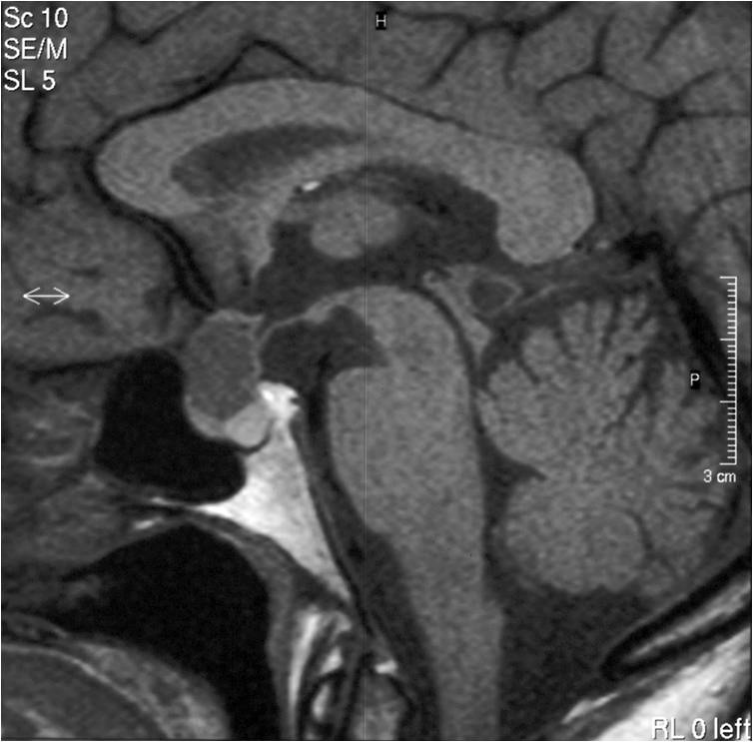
MRI, T1 weighted images, saggital section. A cystic lesion can be seen above the pituitary gland.
